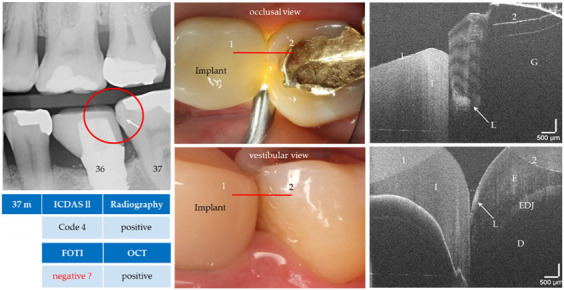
Tooth 37 (m). Apart from the FOTI, the enamel lesion (L, arrows) next to a gold inlay (G) was detected visually, radiographically and by OCT from both the occlusal and vestibular position. The proband was also able to confirm that a non-cavitated lesion had been detected before insertion of the implant crown (I, e.max CAD). The red lines mark the OCT cross-sectional planes. *As a result of focusing on deeper structures, the tooth surface has flipped in the OCT images. Enamel (E), dentin (D), enamel-dentin junction (EDJ). The vertical scales are related to the refractive index n = 1.0 (see remark in Figure 4).*
